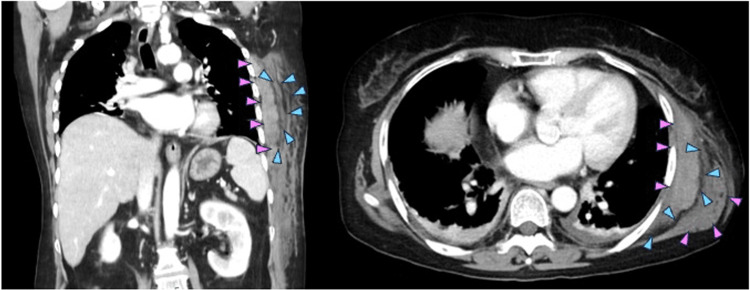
Contrast-enhanced computed tomography of the left lateral chest wall. Contrast-enhanced computed tomography revealed a subcutaneous mass in the left lateral chest wall. This mass was characterized by fluid collection along with fascia of left serratus anterior (blue arrowheads) and swelling of left serratus anterior (pink arrowheads).
